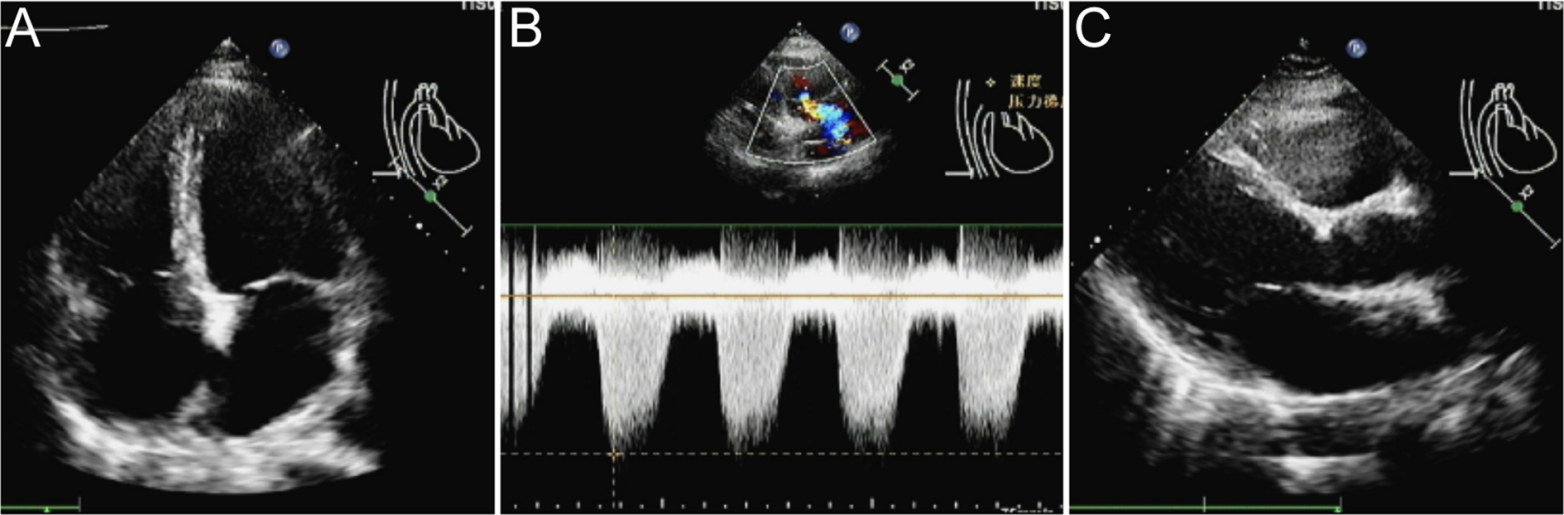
The cardiac ultrasonography. (A) The left atrium was enlarged, with mitral and tricuspid regurgitation. (B, C) The pulmonary artery systolic pressure was 40 mmHg, and the EF was only 44%.
